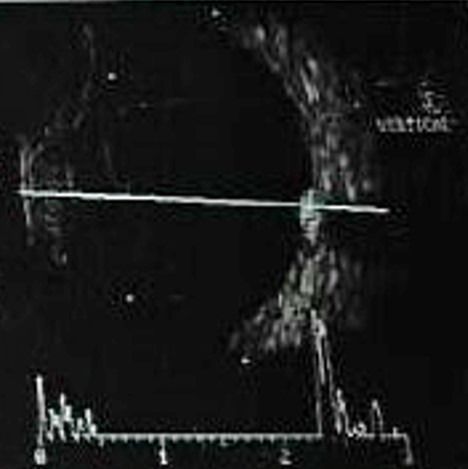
B-scan ultrasonography axial scan showing a dome shaped mass lesion over optic disc with high echogenicity
